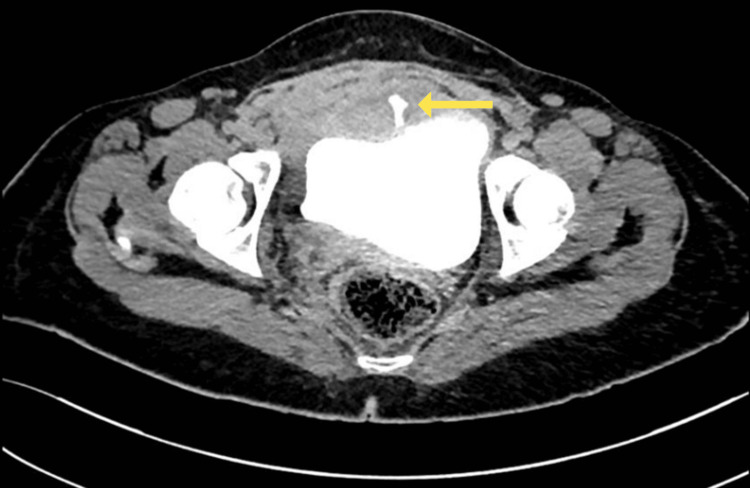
Axial view of the repeated CT cystogram after two weeks from the initial study showing contrast leakage along mid-line of the urinary bladder tear with smaller amount of leakage as compared to the initial scan (yellow arrow). Also noted a partial regression of the previously extraperitoneal collection anterior to the bladder.
